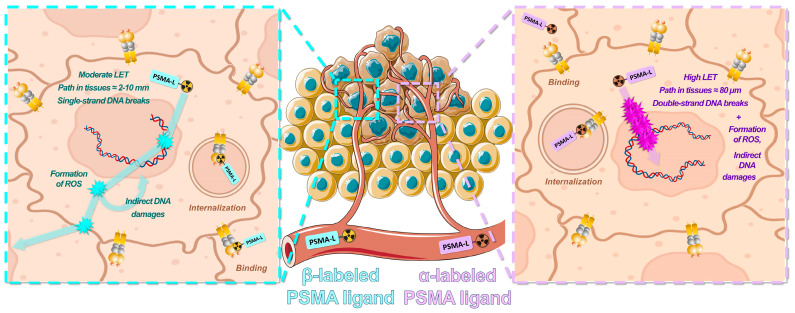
Radiobiology principles associated with PSMA RLT using β-labeled (left) or α-labeled (right) PSMA ligands.
